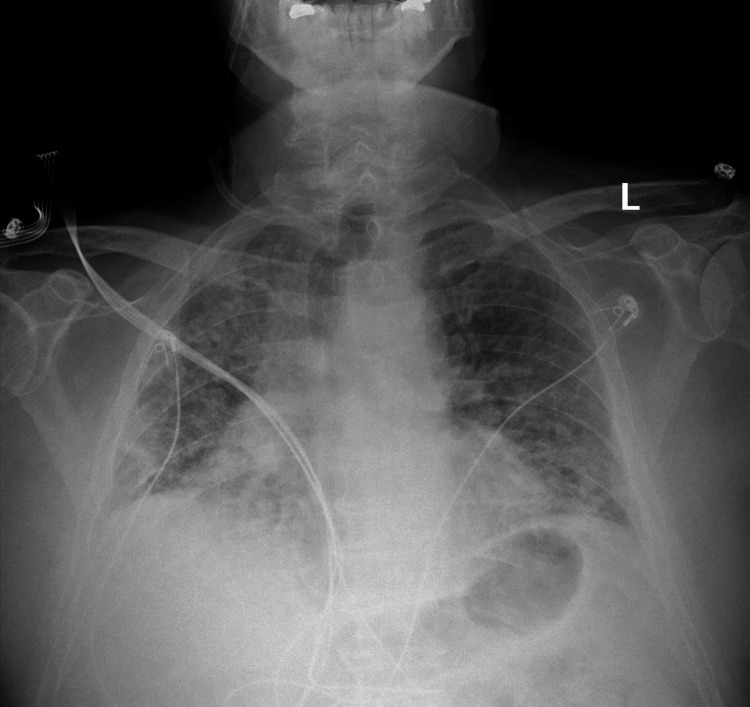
Chest X-ray at week six: diffuse bilateral pulmonic opacities, predominantly interstitial, are once again noted.
